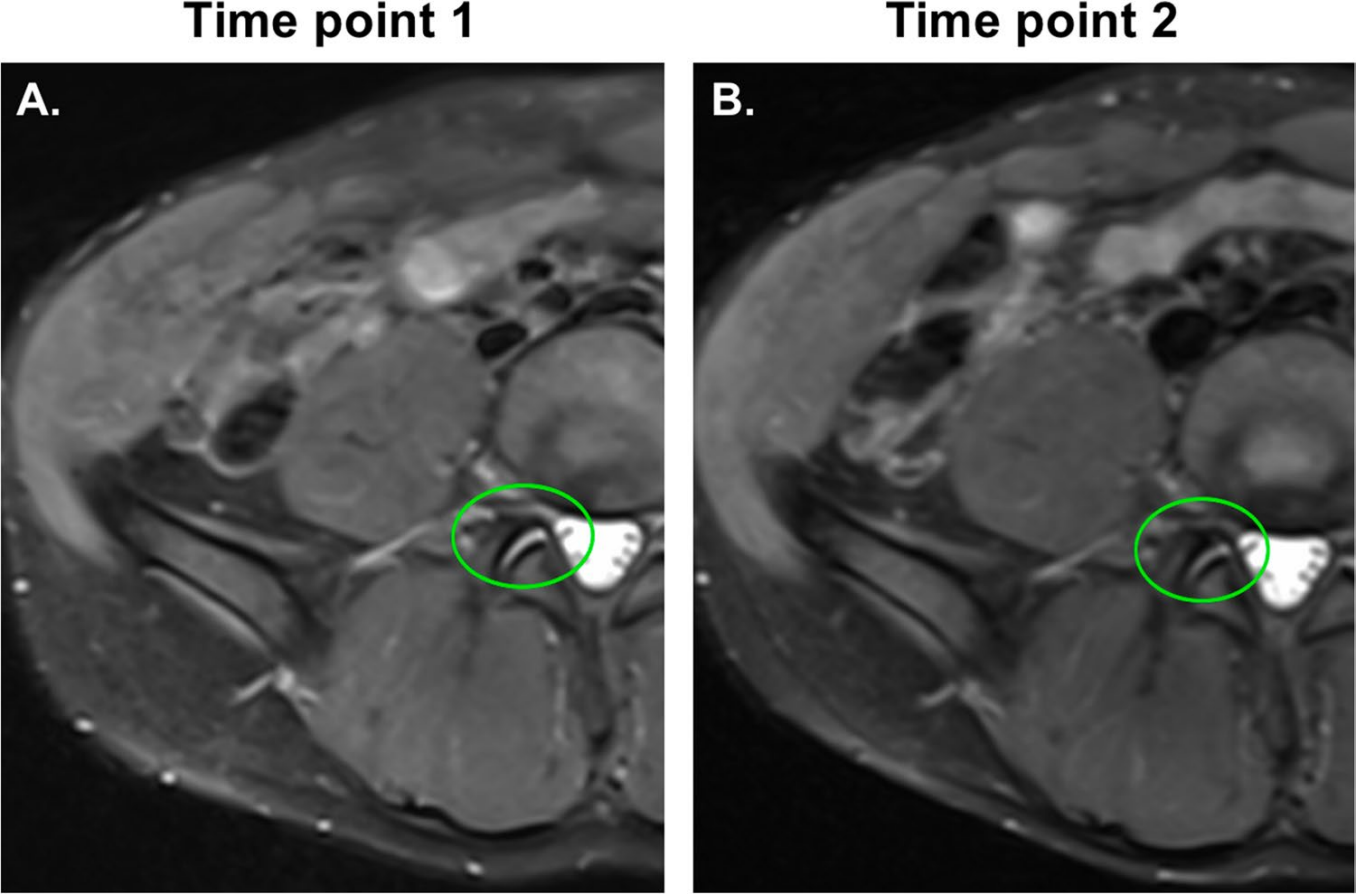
Axial Dixon PD FS TSE MR images of a 35-year-old man showing facet joint effusion at L4–L5 at time point 1 (A) and no worsening at time point 2 (B). No insufficiency fracture at either time point
